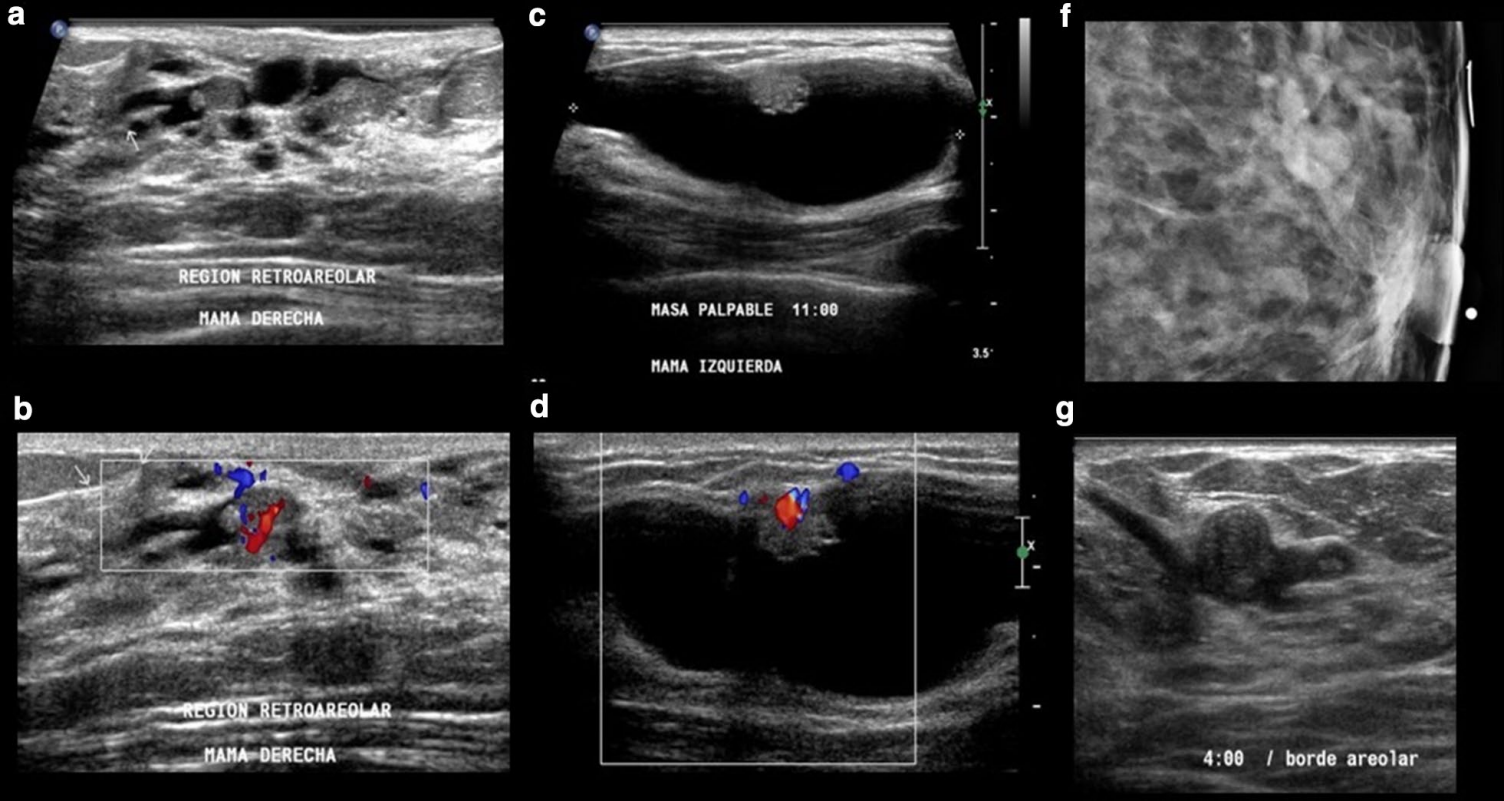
Three different cases of solitary papillomas. 1. a, b Ultrasound shows dilated ducts and an oval and isoechoic intraductal mass with a fibrovascular stem, demonstrated with color Doppler imaging. 2. c, d Ultrasound shows a complex cystic mass with dominant cystic component within a small solid nodule. 3. e Mammography demonstrates a lobular isodense mass in the retroareolar region and (f) ultrasound shows a corresponding intraductal mass
